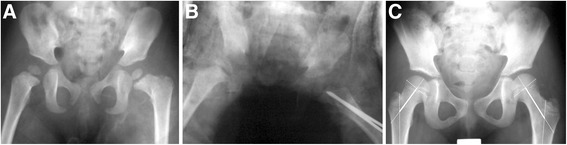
Representative radiographs of femoral neck overgrowth after a type III hip fracture. a A patient with a displaced type III fracture of the left femoral neck. b The two Steinmann pins did not penetrate the growth plate of the injured femoral neck after closed reduction and internal fixation. c Overgrowth (7.5 mm) of the left femoral neck was visible 4 years after injury
